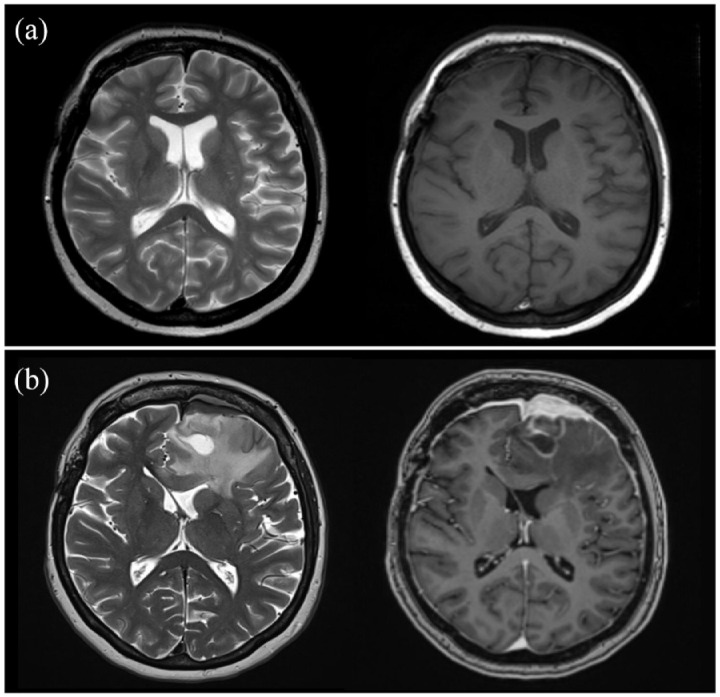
Magnetic resonance imaging findings (a) 14 years earlier and (b) upon admittance.
